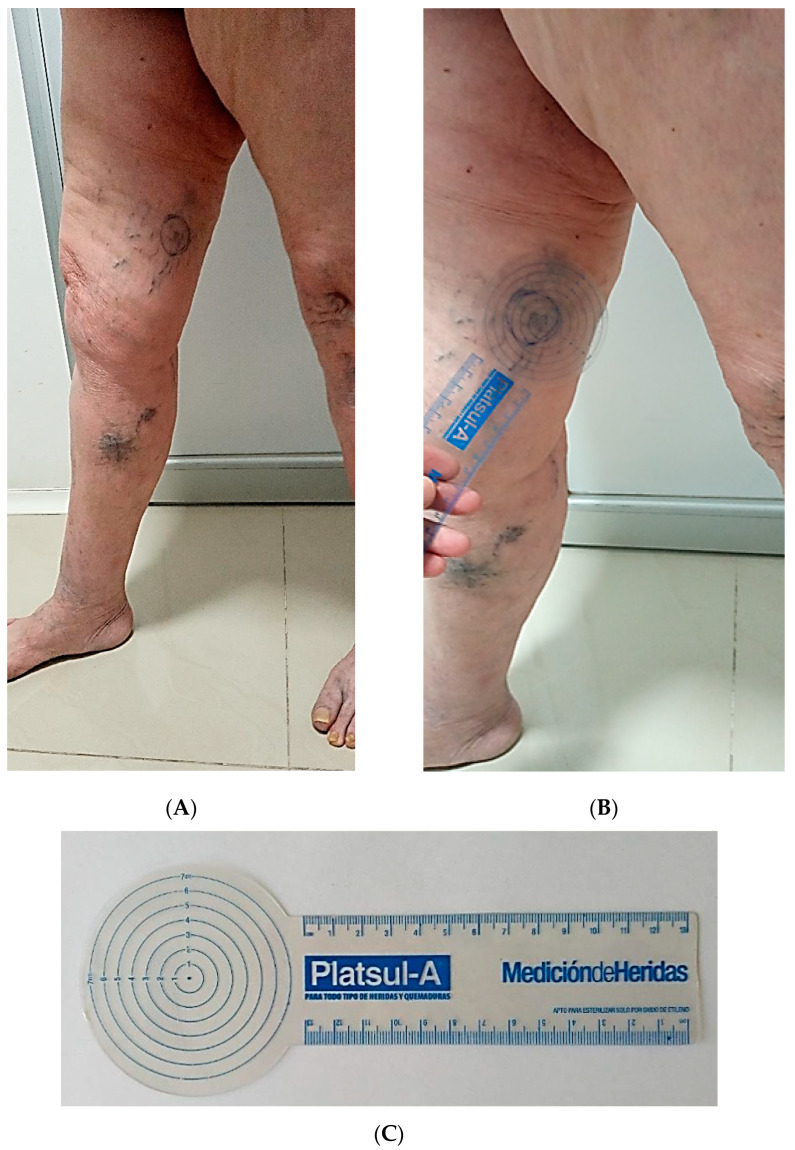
(A) Anatomical outlet site of Hunter’s perforator vein in a woman with CEAP 4 identified by VD. (B) The circle indicates the region of interest (4.0 cm^2^). (C) Instrument for measuring the anatomical region of interest. CEAP: Clinical-Etiological-Anatomical-Pathophysiological classification system. VD: venous Doppler.
